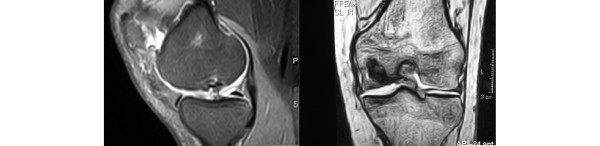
Preoperative magnetic resonance imaging scan of a deep osteochondral lesion. The left image shows the lesion in a sagittal view, eliciting the cartilage damage and the subchondral sclerosis. The right image shows shows the extension of the bone defect in a coronal image.
